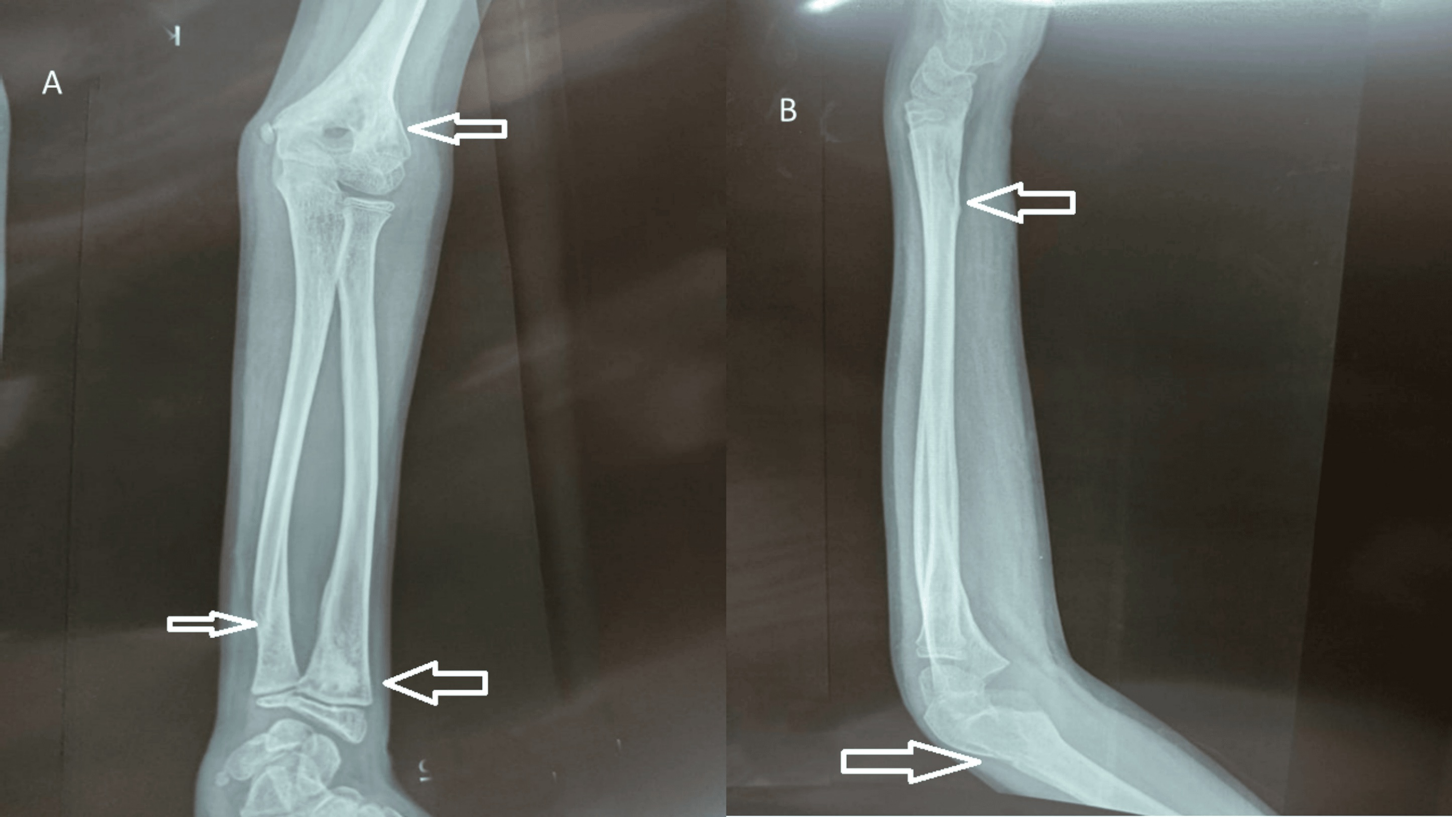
Three-month follow-up radiographs demonstrating complete fracture union and maintained alignment Anteroposterior (A) and lateral (B) radiographs of the left elbow and wrist taken at three months postoperatively showing complete bony union of the supracondylar humerus and distal radius fractures with maintained alignment. The Kirschner wires have been removed, and remodeling is evident at both fracture sites. The patient had regained full, pain-free range of motion without deformity or neurovascular deficit
